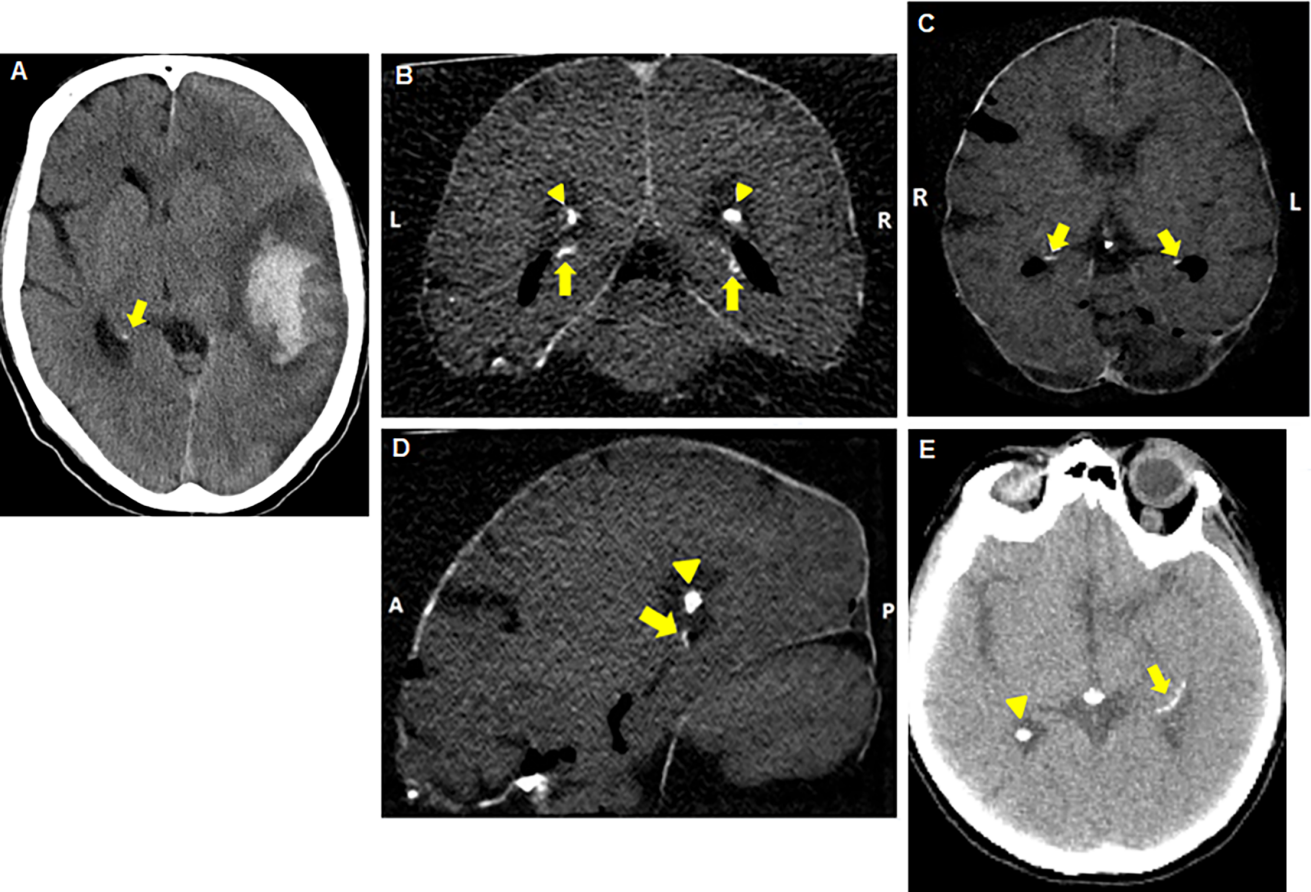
Hippocampal calcifications on CT scans of patient numbers 4 (mild, A), 1 (moderate, B-D) and 3 (severe, E). (A) Axial reconstructed image with mild hippocampal calcification (one dot). (B) Coronal reconstructed image shows (moderate) bilateral hippocampal calcification (multiple dots), indicated by arrows. Choroid plexus calcification is indicated by arrowheads. (C) Axial reconstructed image with moderate bilateral hippocampal calcification marked with arrows. (D) Sagittal reconstructed image with moderate hippocampal calcification marked with an arrow and choroid plexus calcification marked with an arrowhead. (E) Axial reconstructed image shows severe hippocampal calcification (confluent) indicated by an arrow and calcification of the choroid plexus is indicated with an arrowhead.
